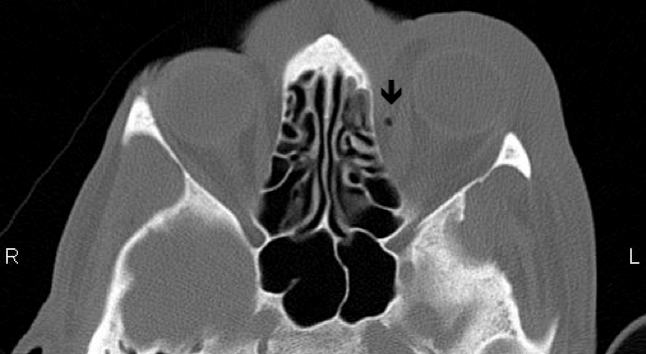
Transverse section of MRI scan showing air locule
